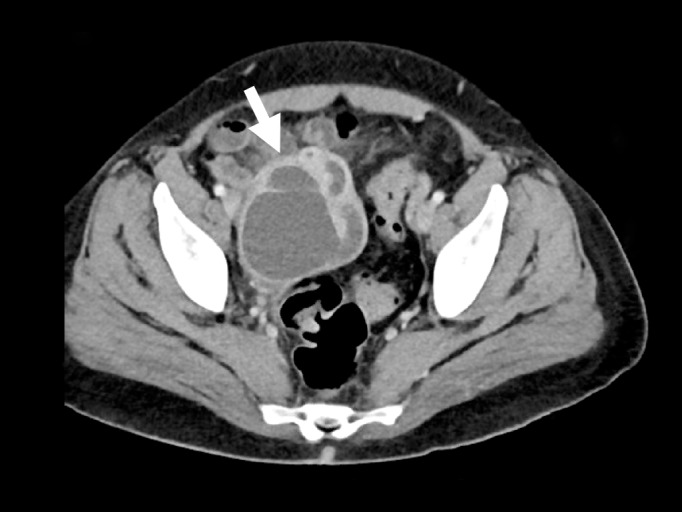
The computed tomography finding which confirmed the diagnosis.
